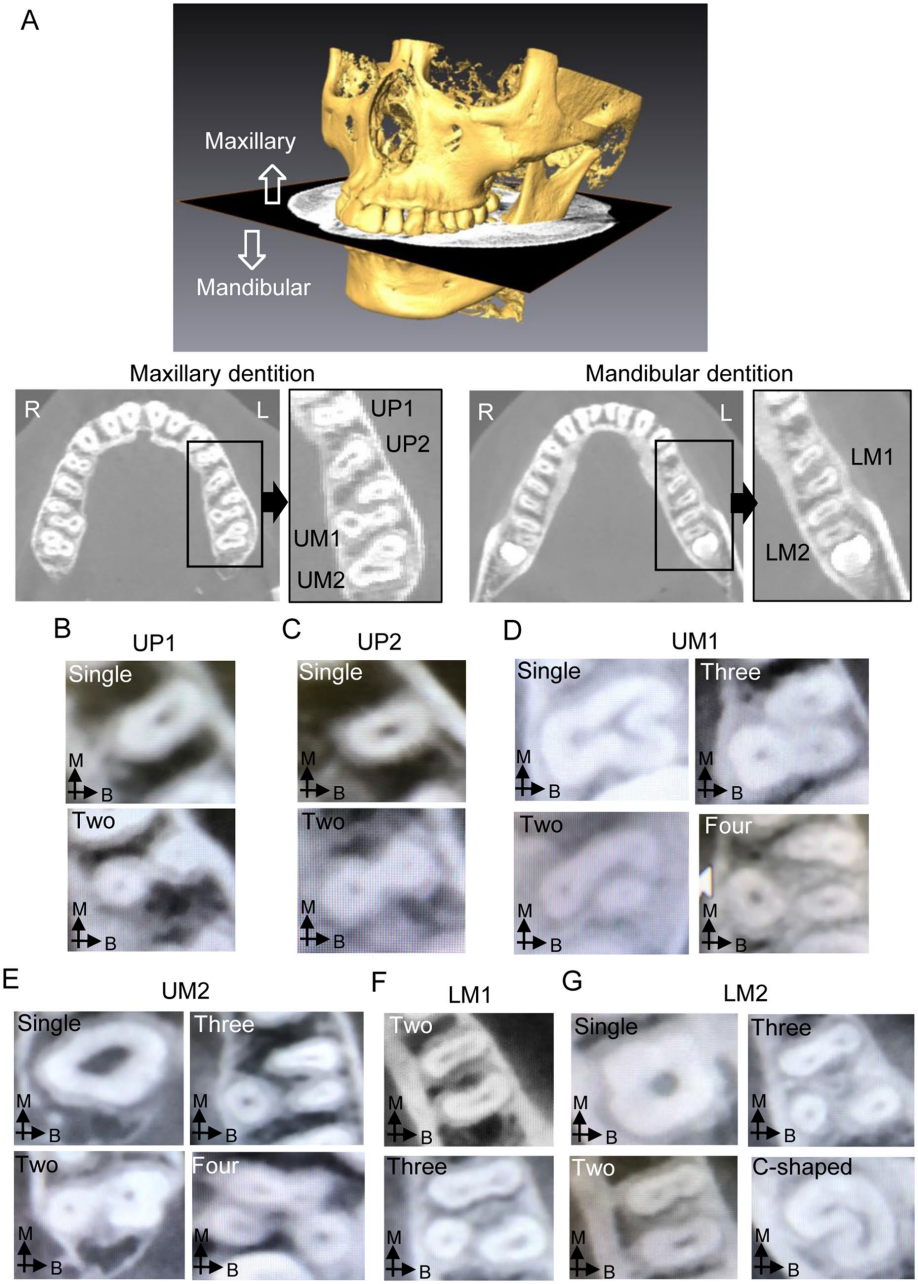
Observation of tooth root morphology using CT. (A) Reconstruction of the three-dimensional surface and the occlusal plane (OP) using Amira ver 6.0.0. The number and shape of roots in the maxillary and mandibular teeth were observed in slice images parallel to the OP. (B–G) Examples of the tooth root morphology observed in UP1s (B), UP2s (C), UM1s (D), UM2s (E), LM1s (F), and LM2s (G). The arrows indicate the buccal (B) and mesial (M) directions.
